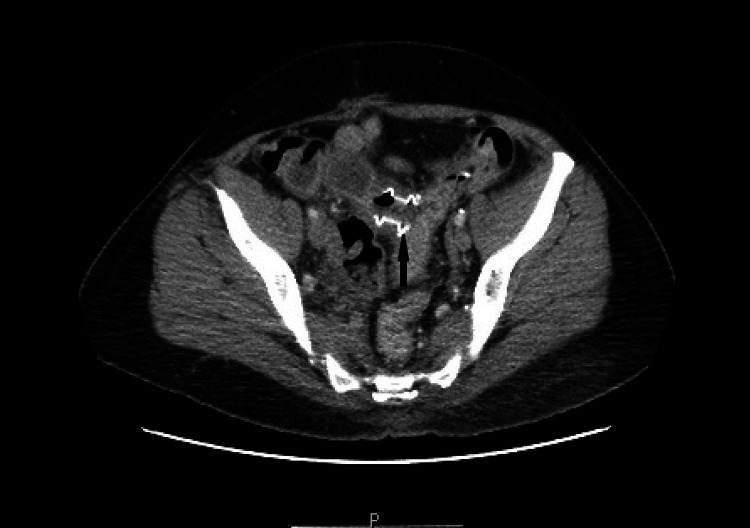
Computed tomography of the abdomen showing the AXIOS stent (arrow) at the ileosigmoid stricture relieving the obstruction
